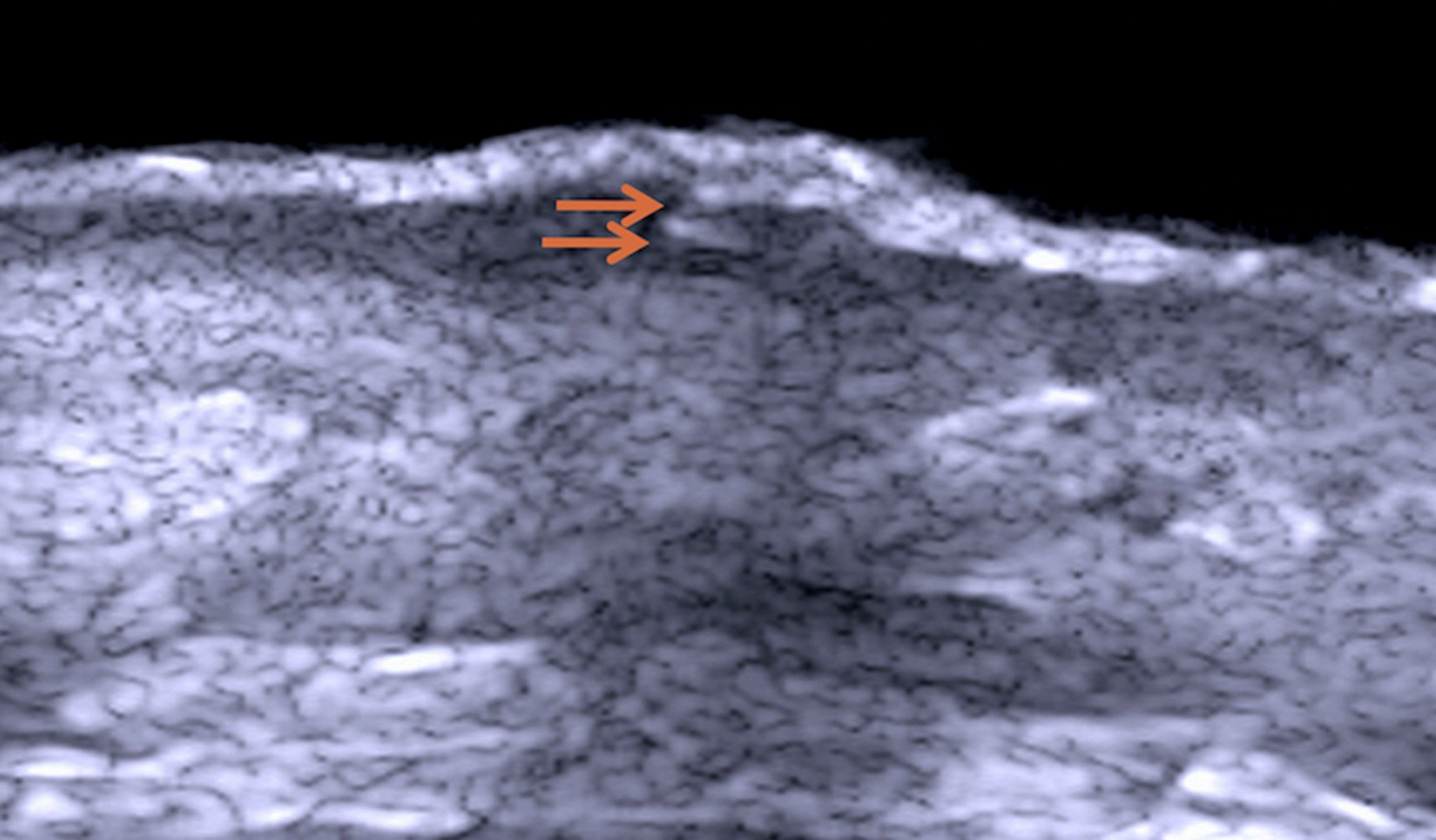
High-frequency ultrasound with Doppler shows epidermal thickening, hyperechogenic round structures (arrow), dermal fibrosis, and increased vascularization. My Lab Touch, 22 MHz linear transducer.
